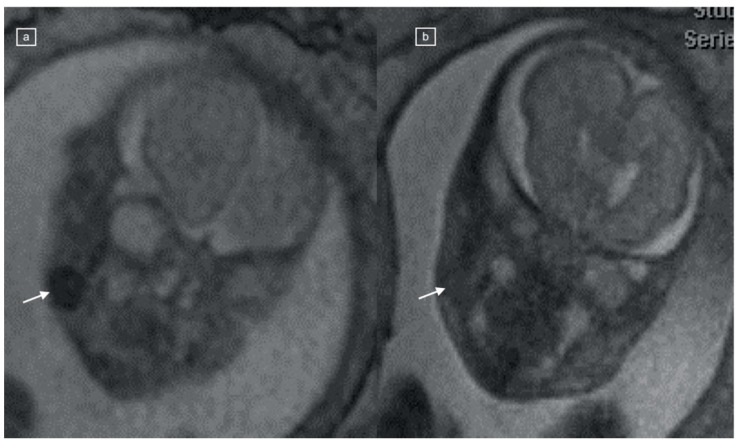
(a,b) Comparison of TRUFI and HASTE MRI appearances of the fetal buccal fat pad in a 25-week fetus with congenital diaphragmatic hernia. (a) Coronal TRUFI demonstrates the oval low-signal-intensity fat pad on the right (arrow), which is isointense to soft tissue on the HASTE image (b).
